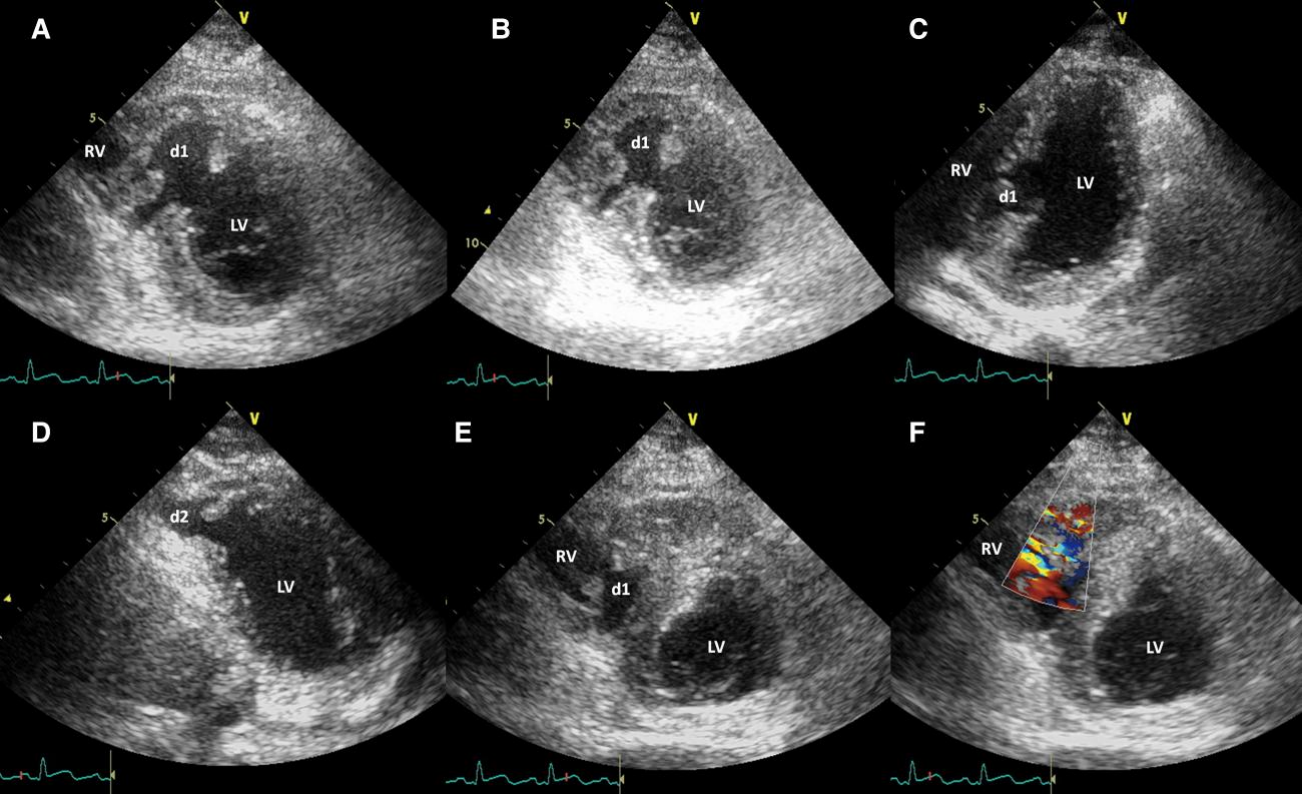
Echocardiography demonstrating an interventricular septal myocardial dissecting tear (d1) and rupture into the left and right ventricles (*A–C*) and an apical myocardial dissecting laceration (d2) and rupture into the left ventricle (*D*). An interventricular septal dissecting rupture with a small left-to-right shunt is demonstrated (*E* and *F*). LV, left ventricle; RA, right atrium; RV, right ventricle.
